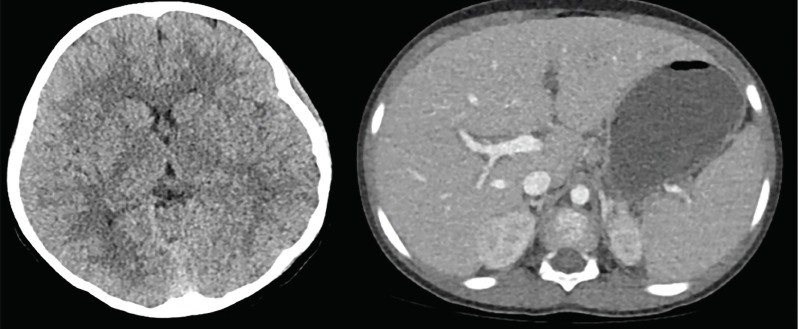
Computed tomography of the head (left) and abdomen and pelvis (right).
